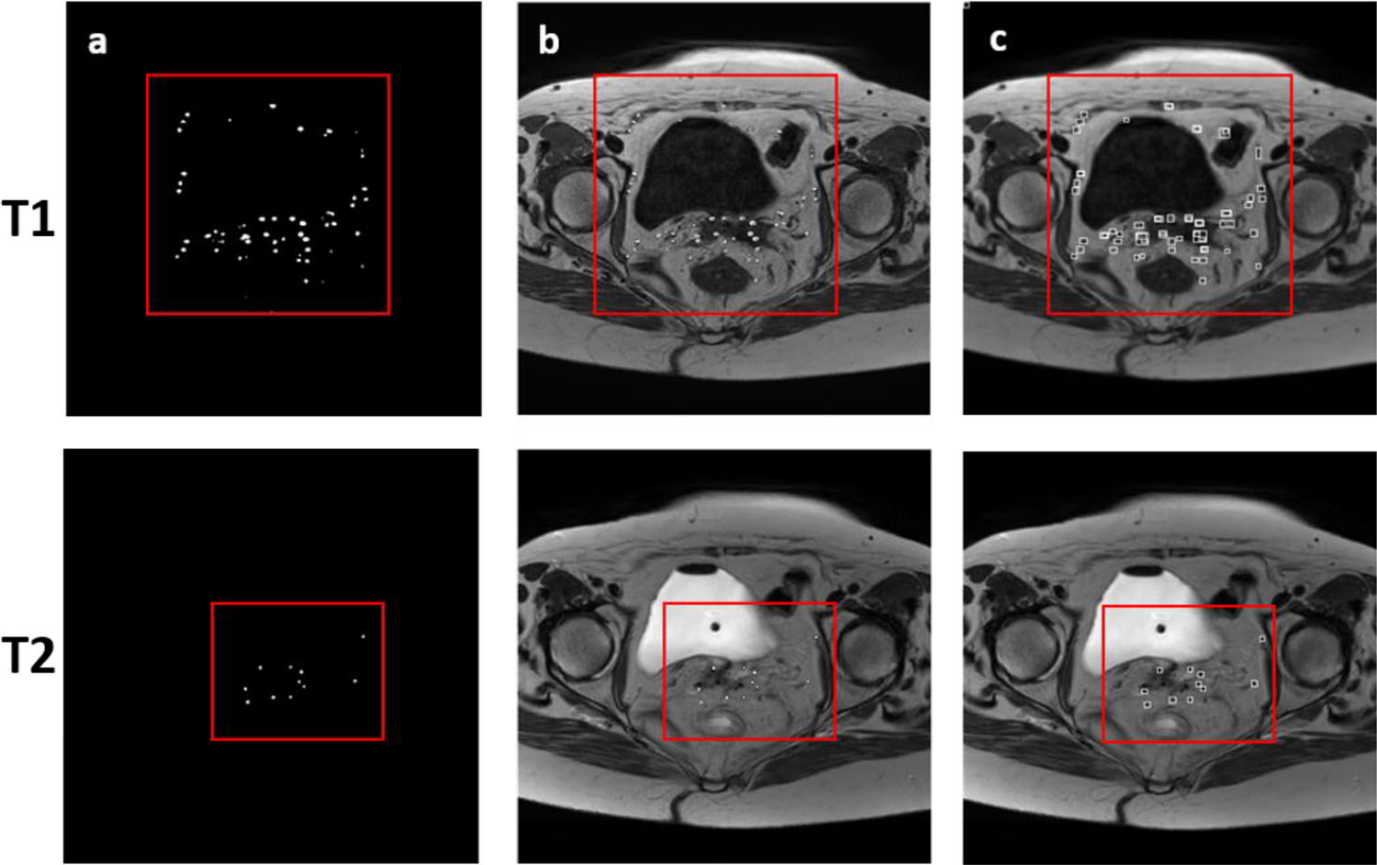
Algorithm postprocessing step using magnetic resonance imaging (MRI) images of one gynecological patient (testing set). (a) The red box shows the U‐net output (true and false positive catheter positions); (b) using the U‐net output approximate positions, catheters were located in the MRI images, T1w(top) and T2w (bottom) images, respectively; (c) rectangles were then calculated and used to find the exact positions of catheters in both image sets. As a final step, hyperintense (T1W) and hypointense (T2W) regions within those rectangular are localized as catheters
